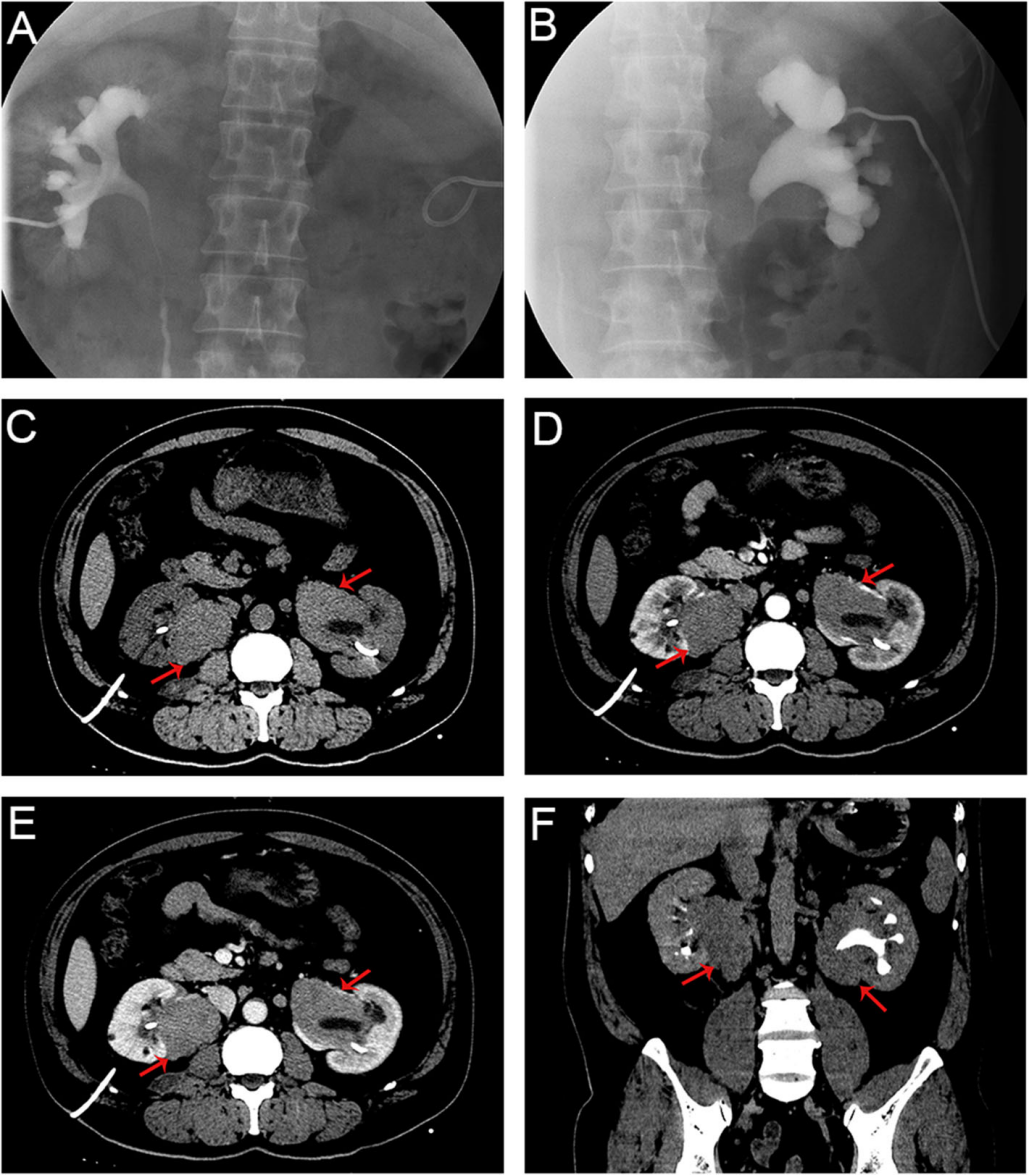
The antegrade urography of the left (A) and right (B) kidney, and contrast-enhanced abdominal CT urography of the lesions (red arrow) during plain scan phase (C), arterial phase (D), venous phase (E) and delayed phase (F) in case 2
